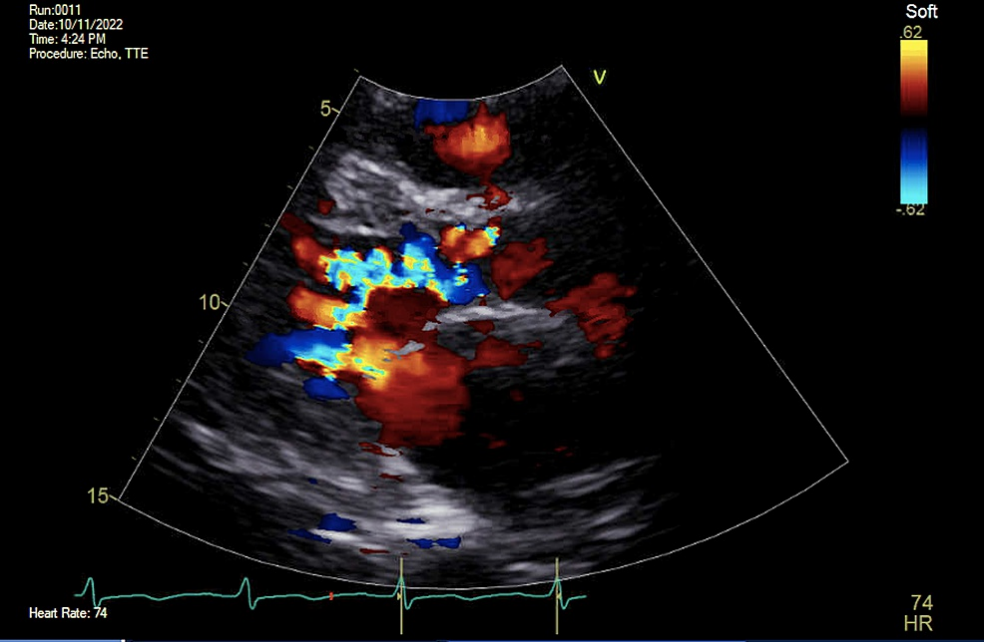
Transthoracic parasternal long echocardiogram view with Doppler demonstrating regurgitant flow from the aortic valve
